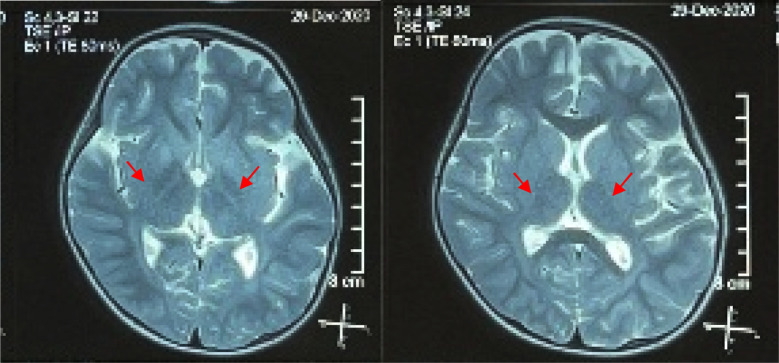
The second head MRI showed a white matter lesion at globus pallidus bilateral that supports the encephalopathy metabolic view (new lesion), subdural fluid collection on bilateral frontal regions, focal brain atrophy fronto-temporo-parietal bilateral lobe, cerebellum, and brain stem (increasing process), and microcephalic with brachycephalic skull type.
